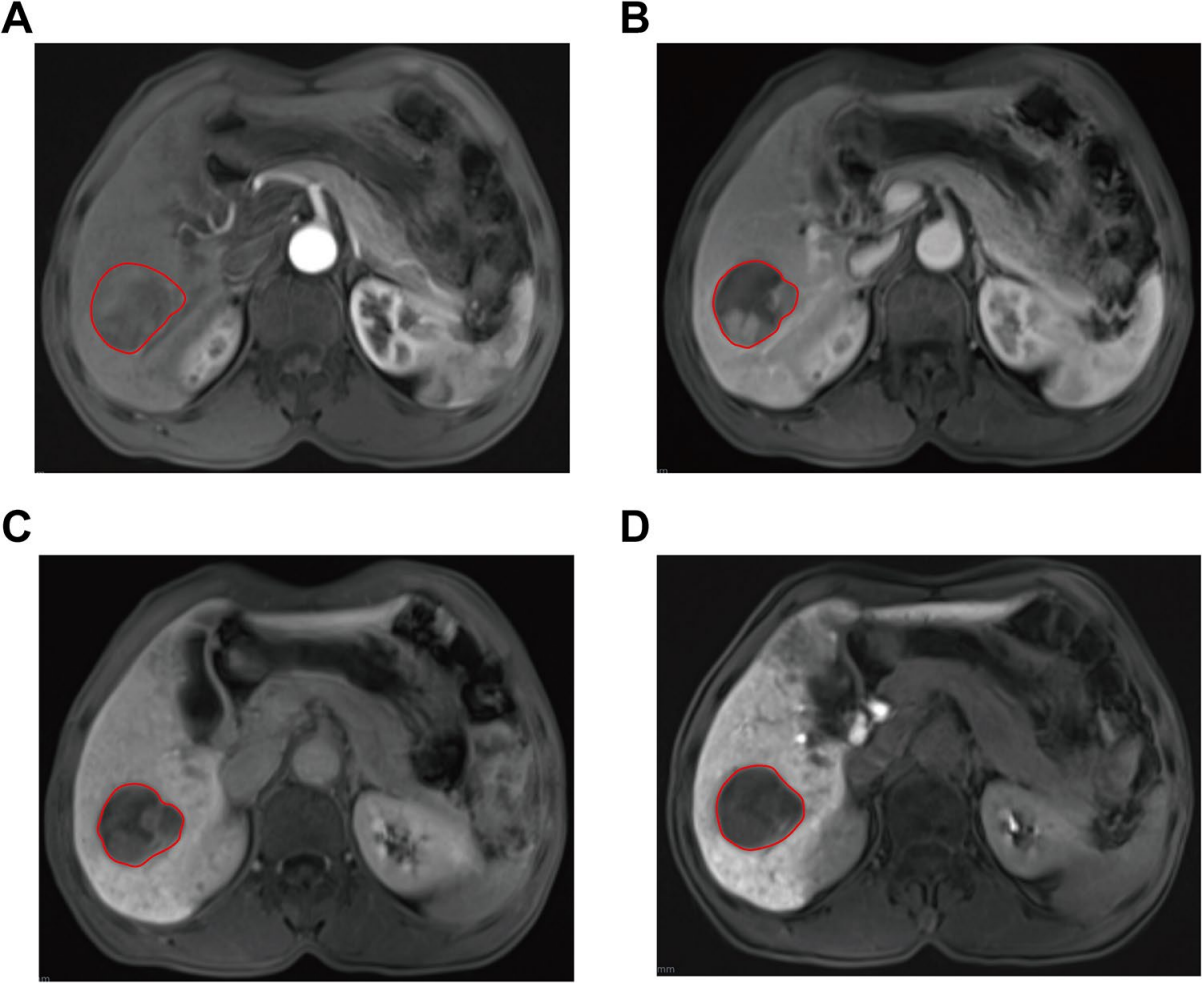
MRI images of arterial phase (A), portal venous phase (B), delayed phase (C) and hepatobiliary phase (D) of a 59-year-old male patient and delineation of region of interest.
